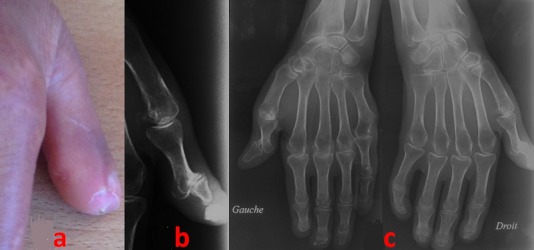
A) thickening and tightening of the skin over the thumb with deposition of calcific nodules; (B) radiographs of her thumb showing a sub-cutaneous calcification; (C) acro-osteolysis in the hands X-rays
